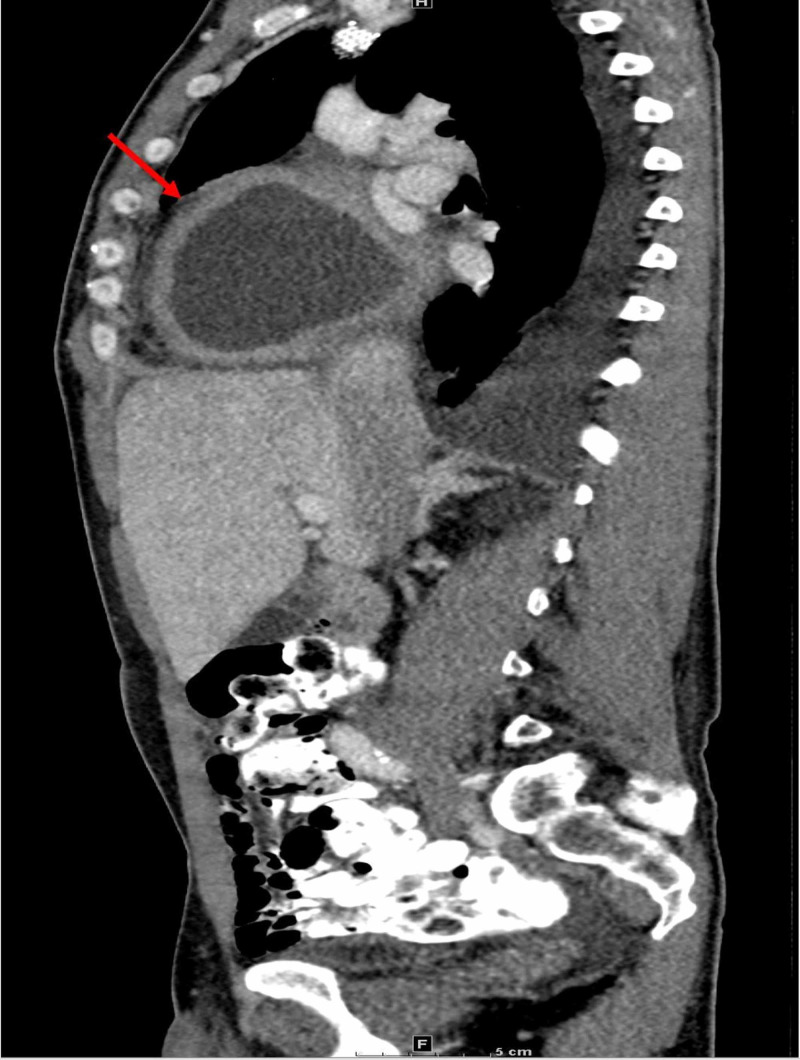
Pericardial Abscess: Sagittal CT Chest, Abdomen, and Pelvis With IV and Oral Contrast.
